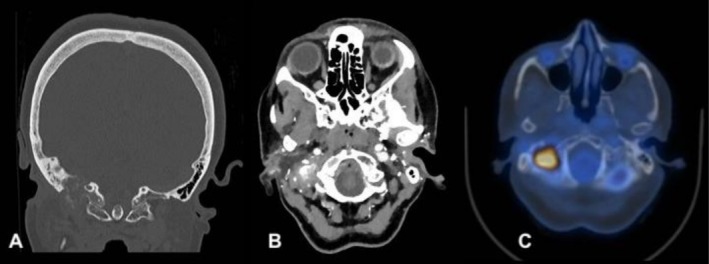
Expansive lesion at the external auditory canal (23 × 20 × 12 mm), localized to the right posterior foramen lacerum, with osteolysis of the rocca petrosa (A), indissociable from the internal jugular vein (B), and with an increased FDG uptake (SUV max 21.4) at the PET/TC scan (C).
